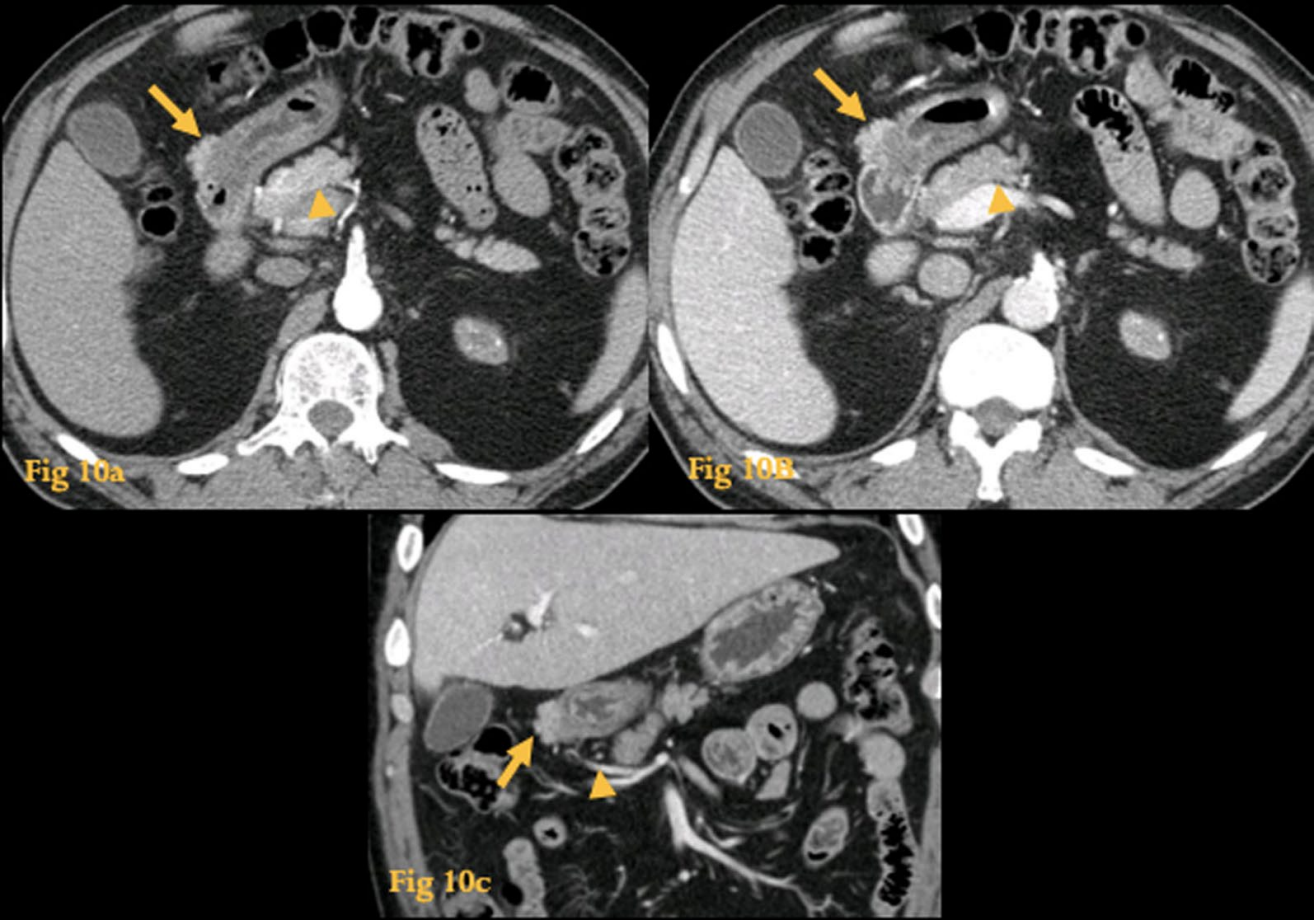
Ectopic pancreas. Abdominal CT of a patient who underwent the exam for an unrelated reason. A soft-tissue nodule, with a flat-ovoid shape and microlobulations, is seen in the serosa of the first portion of the duodenum. It has an enhancement pattern similar to that of the normal pancreatic tissue (arrowheads on a, b) in the late arterial (a) and portal venous phases (b). The lesion (arrow) is supplied by a branch of the gastroduodenal artery (arrowhead on c). This was heterotopic pancreatic tissue in the duodenal bulb serosa
